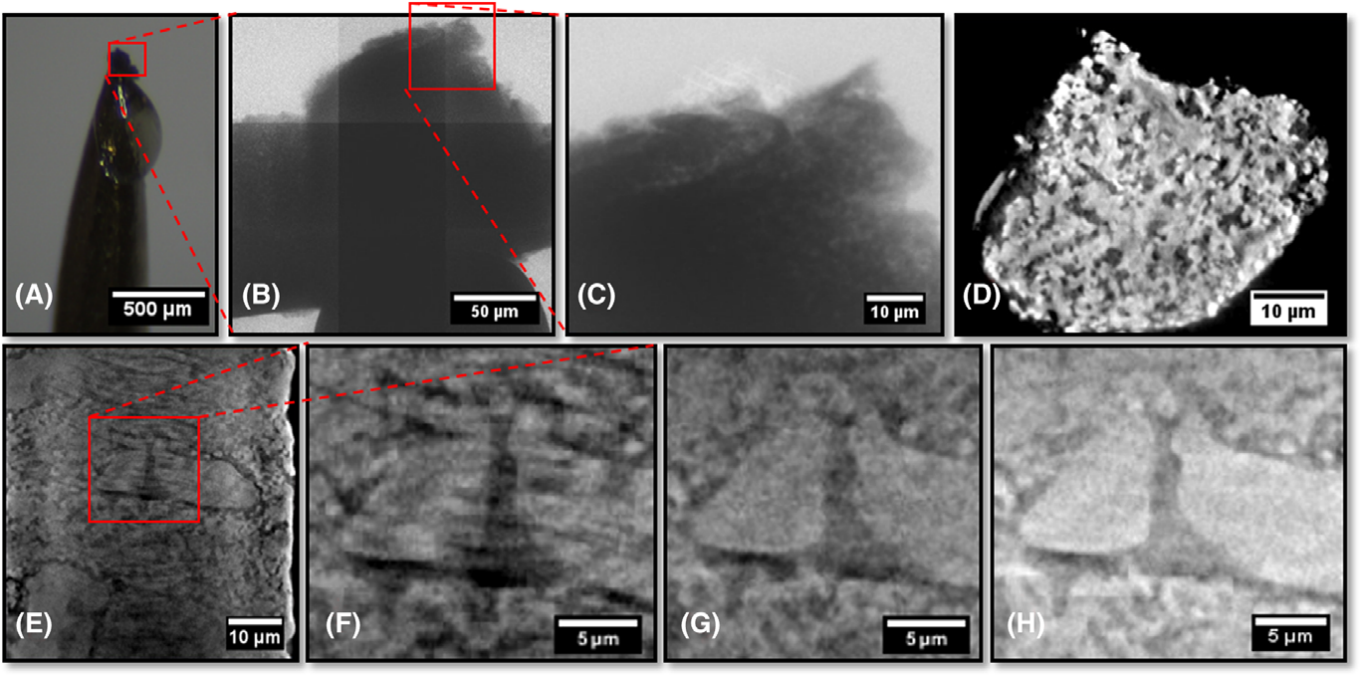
(A) Optical image of traditional mechanical preparation where the red square highlights the location of the sample material. (B) Corresponding mosaic of radiographs of the sample material. (C) Single radiograph with a FOV equal to that used for CT. (D) Single raw orthoslice from reconstructed tomogram. (E) Tomographic vertical slice from 60 μm pillar, magnified features at (F) 901 projections, binning 2, (G) 1601 projections, binning 1 and (H) 1601 projections, binning 2.
